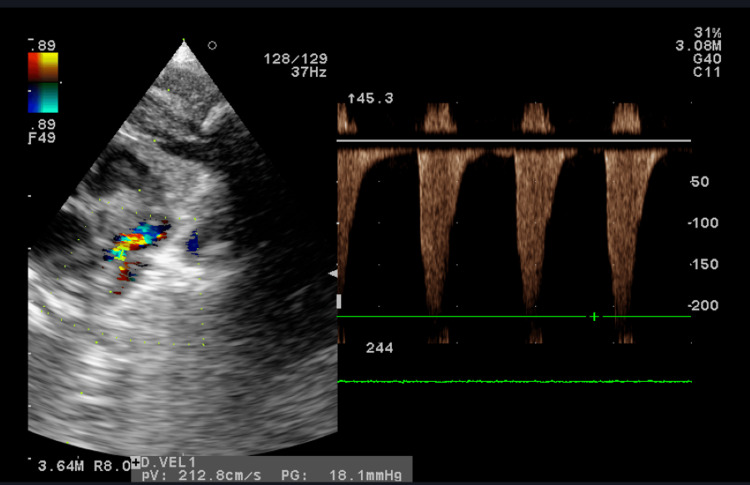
Echocardiography (Eco 2D/ Doppler) on day 3 (Case 1): turbulence distal to the subclavian artery with a gradient of 18 mmHg, without CoA flow, and a closed ductus arteriosus. CoA: coarctation of the aorta
